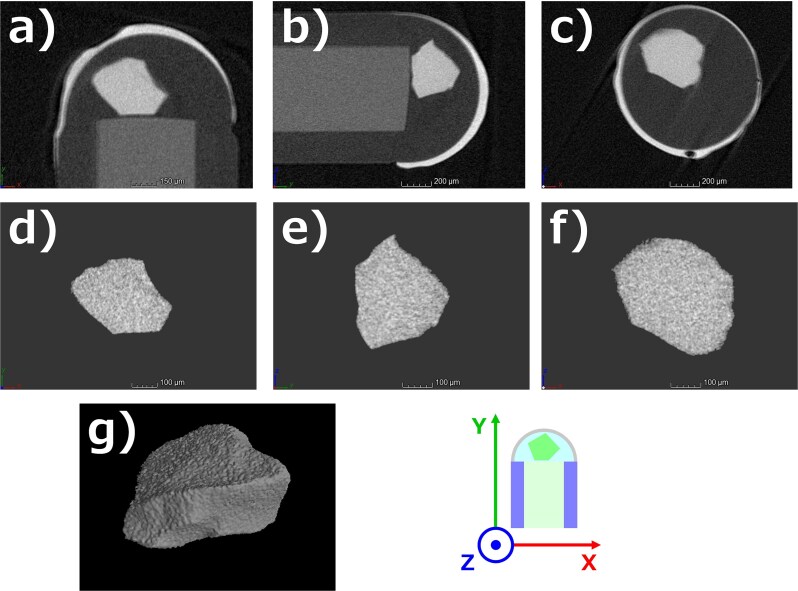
Examples of the reconstructed CT image as a 2D cross-section for the (a) x–y, (b) y–z, and (c) z–x planes, and the extracted VOI as a 2D cross-section for the (d) x-y, (e) y–z, and (f) z–x planes, and (g) 3D reconstructed volume obtained for sample ‘En-L’.
